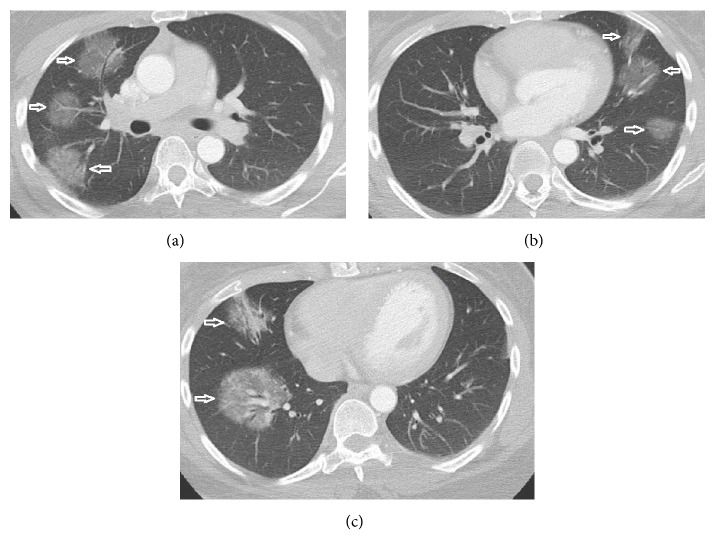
Axial contrast-enhanced CT obtained at different levels (a, b, c), demonstrating bilateral mass-like ground-glass opacities in a peribronchovascular distribution (arrows) with air-bronchograms and vessels seen coursing through.
